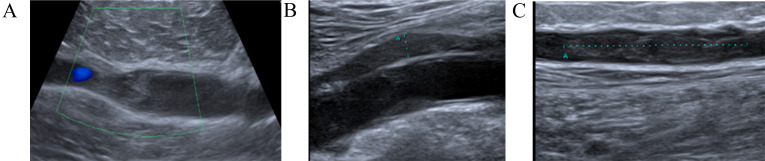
Dynamics imaging with compression ultrasound (CUS). (A) Right lower extremity CUS
